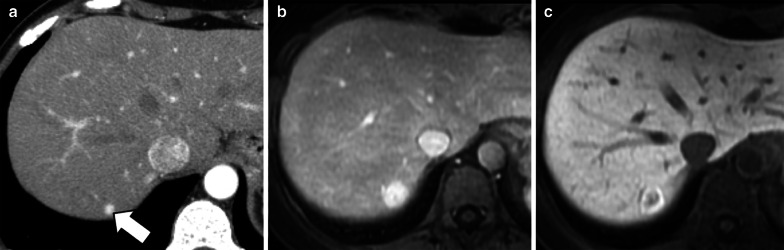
A 40-year-old woman with sigmoid adenocarcinoma and liver metastases treated with chemotherapy (XELOX regimen). a Contrast-enhanced CT shows a FNH-like nodule (arrow) that is hypervascular in the arterial phase. Gadoxetate disodium-enhanced MRI performed 2 years later (b) in the arterial phase and (c) hepatobiliary phase shows size increase which raises the suspicion of metastasis. However, the hepatobiliary phase (c) demonstrates a hyperintense rim establishing the diagnosis of FNH-like nodule
